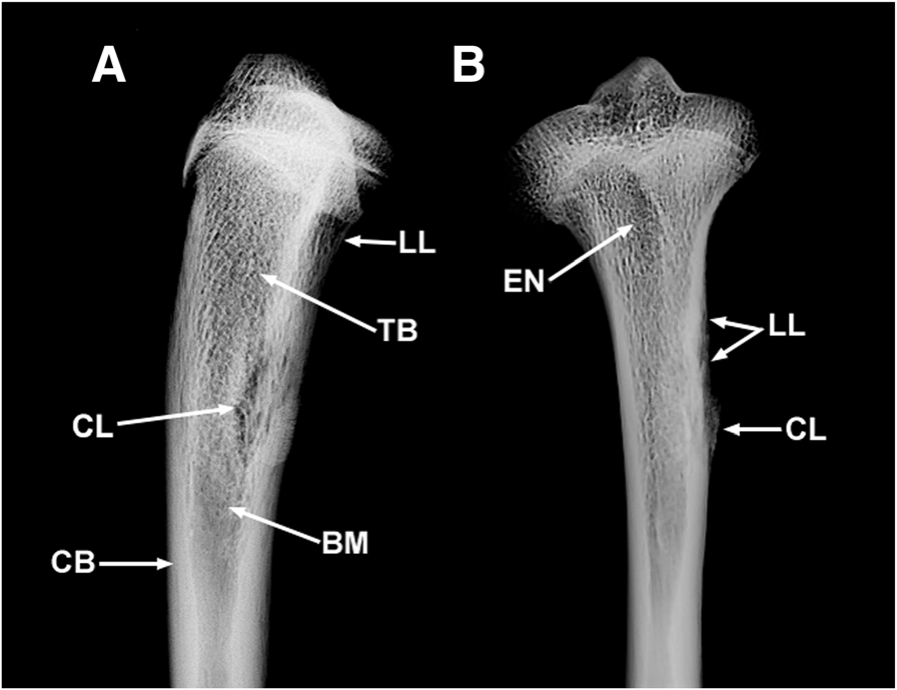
Radiographs of the injured right tibia of *Tamandua tetradactyla* (MN 79028). a Right tibia in medial view; b Right tíbia in anterior view. BM, Bone marrow; CB, Cortical bone; CL, Cloacae of pus; EN, Enthesopathy; LL, Lytic lesion; TB, Trabecular bone
